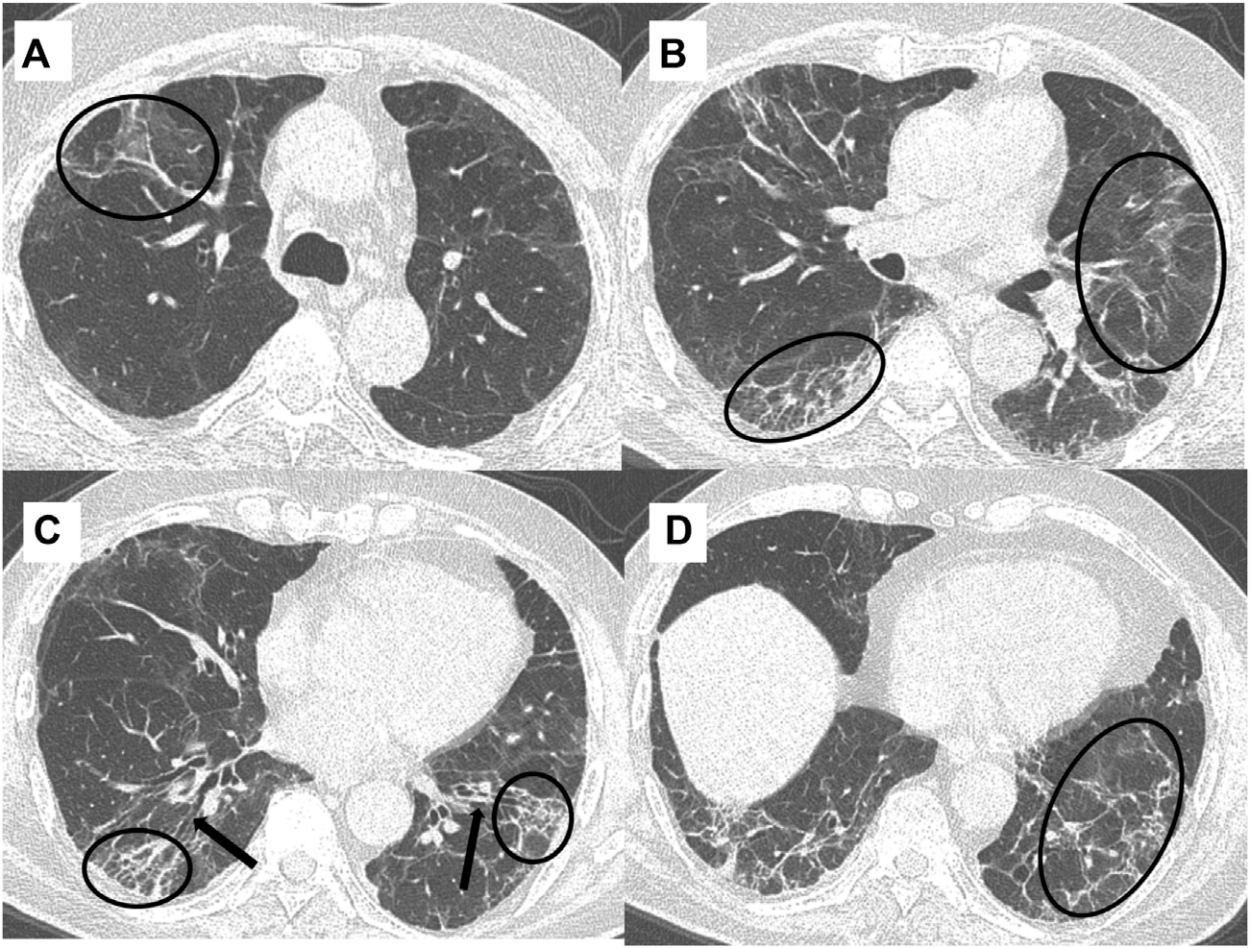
A 49-year-old, COVID-19 ARDS recovered male patient presented with persistent cough and mMRC class 3 dyspnea at six-month follow-up. Spirometry showed an FVC of 60% and high-resolution chest CT (HRCT) axial sections (A–D) showed the presence of reticulations/septal thickening and parenchymal bands (black circles), and traction bronchiectasis (black arrows) interspersed with patchy areas of background GGOs.
